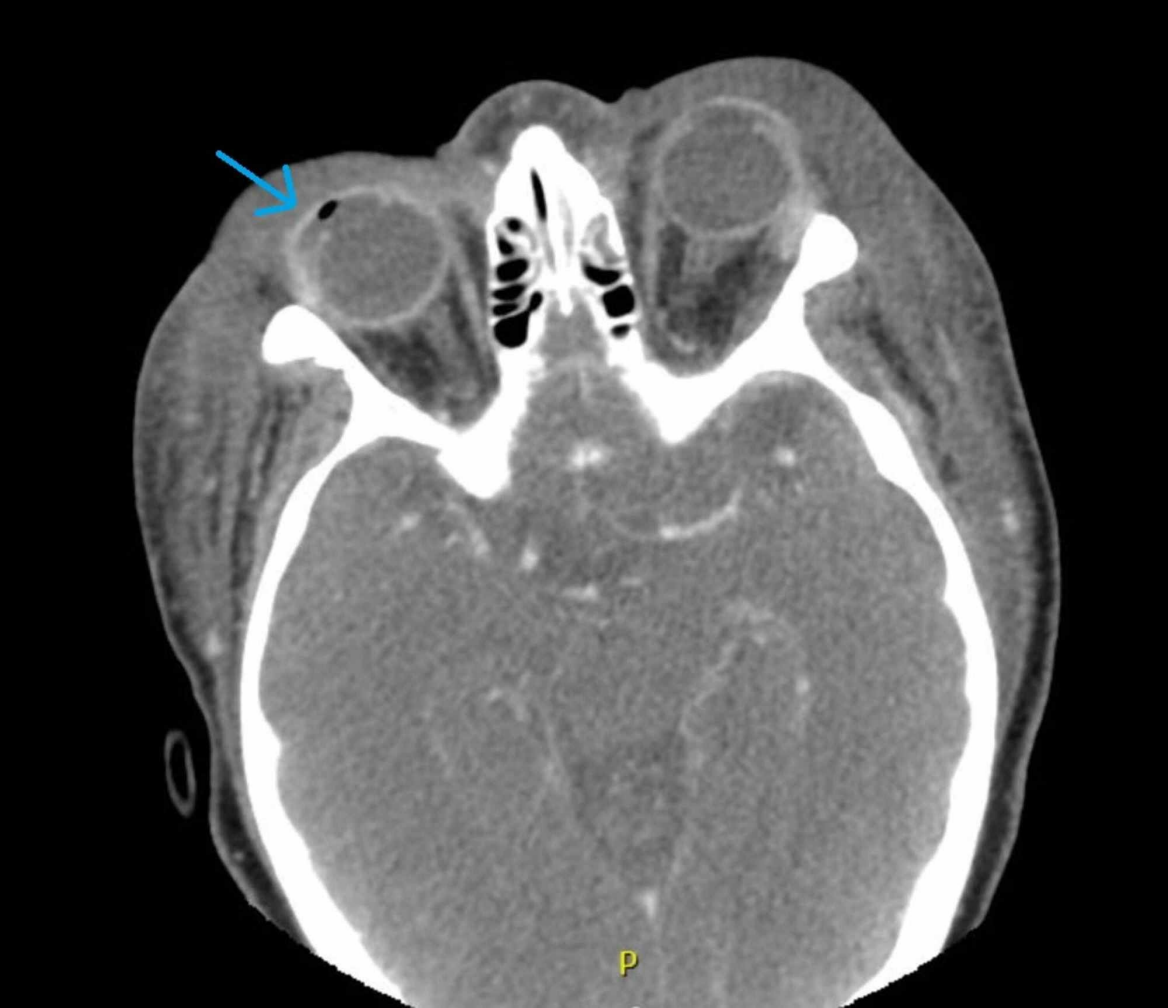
Axial CT imaging shows bilateral periorbital inflammation with right-sided abscess and emphysema
